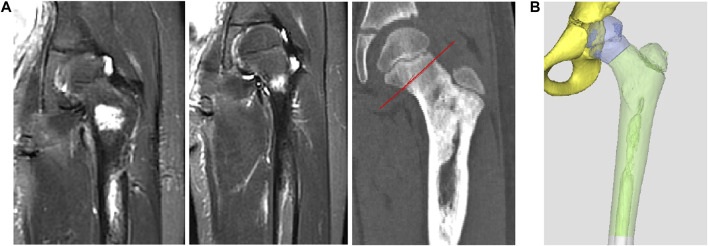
Preoperative osteotomy design planning. (A) The determination of osteotomy by MRI. (B) The design of preliminary prosthesis by software.
